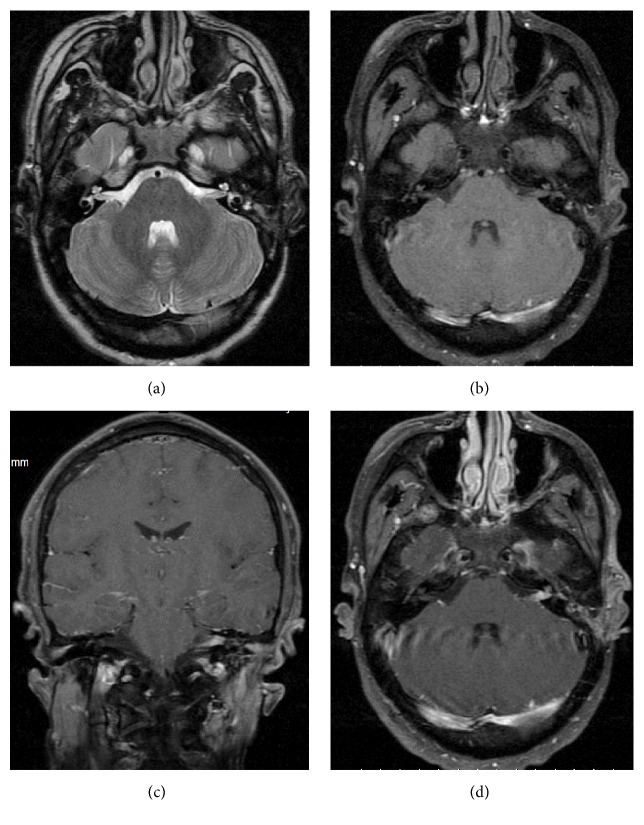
A 25-year-old man with rhabdomyosarcoma of the left cheek. Selective involvement of the left complex of cranial nerves VII and VIII. MRI; FSE/T2WI (a); fat-saturated T1WI before (b) and after gadolinium administration (c, d).
